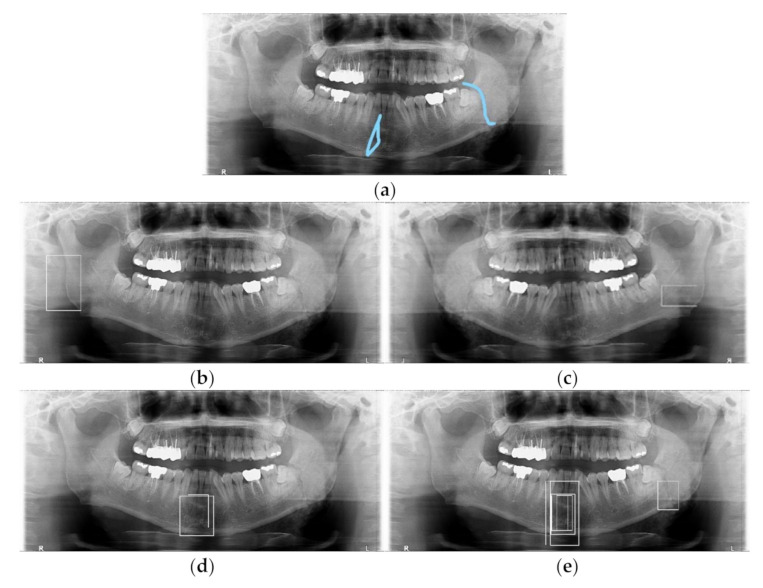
The comparison between different four modules for mandibular fracture detection (white boxes): (a) Diagnosed radiographs (blue lines) by a radiologist, (b) Original module, (c) Gamma modulation module, (d) Luminance adaptation transform module, and (e) The proposed module.
